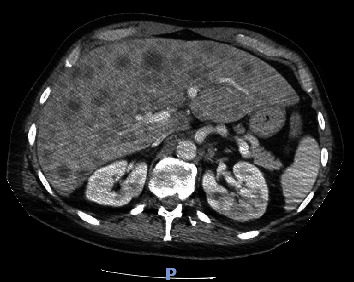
Axial section obtained from computed tomography of the abdomen and pelvis shows numerous hypovascular liver masses measuring up to 4.8 cm.
